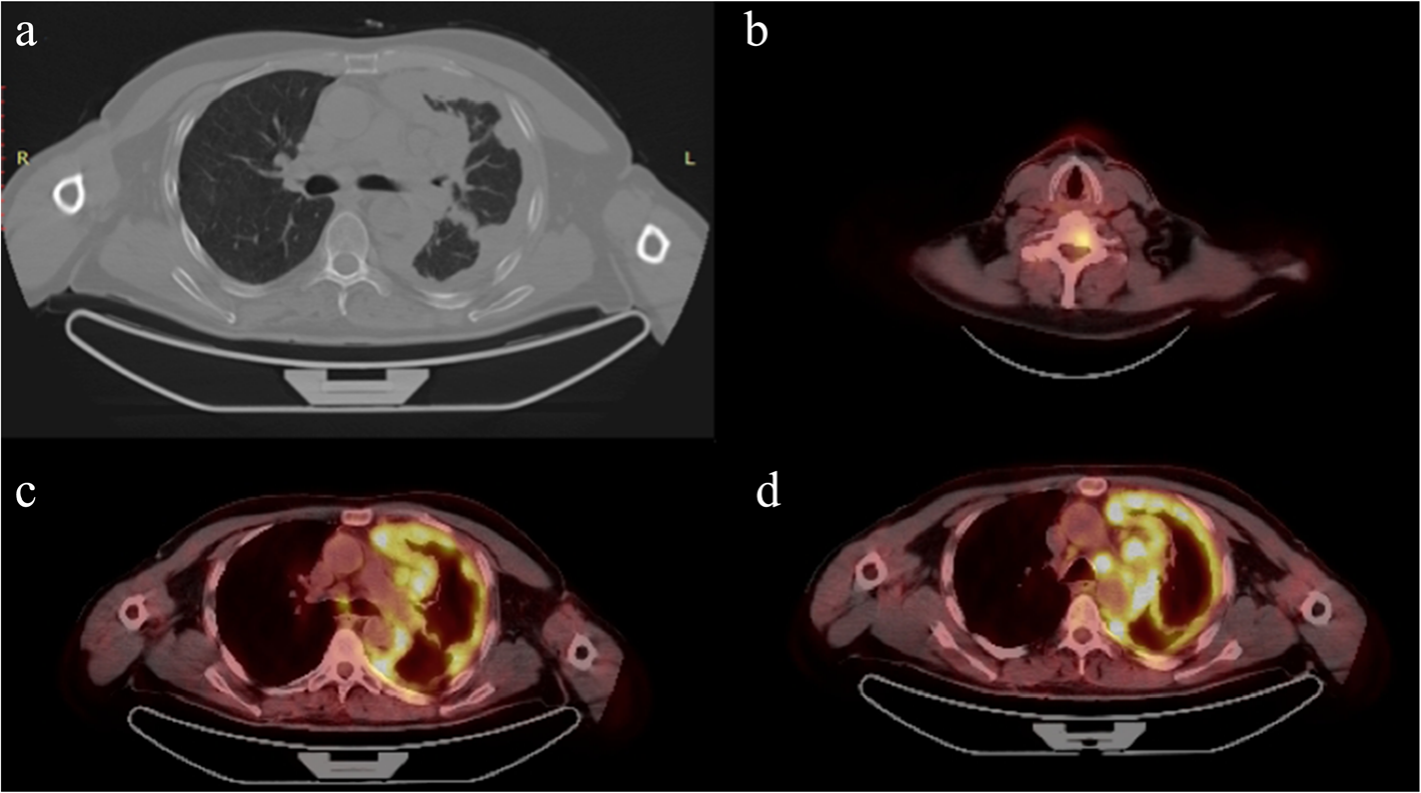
a Chest CT presenting mesothelioma. b, c Axial PET images with higher tracer uptake. d Axial PET image indicating C7 vertebrae having a possible metastatic view
